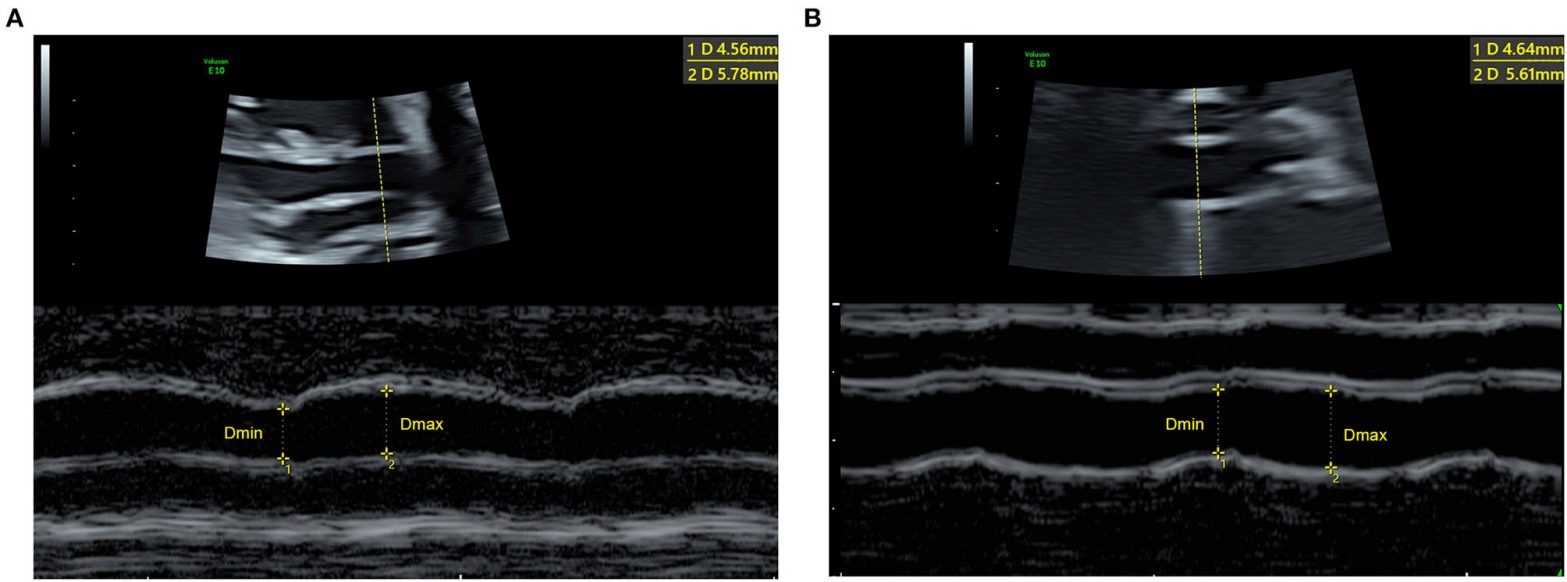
Measurement of the maximum and minimum internal aortic diameters to assess aortic strain from the M-mode echocardiography of a 32-week normal fetus (A) and a 33-week fetus with CoA (B). Dmin, the minimum internal diameter in the diastolic phase, Dmax, the maximum internal diameter in the systolic phase.
